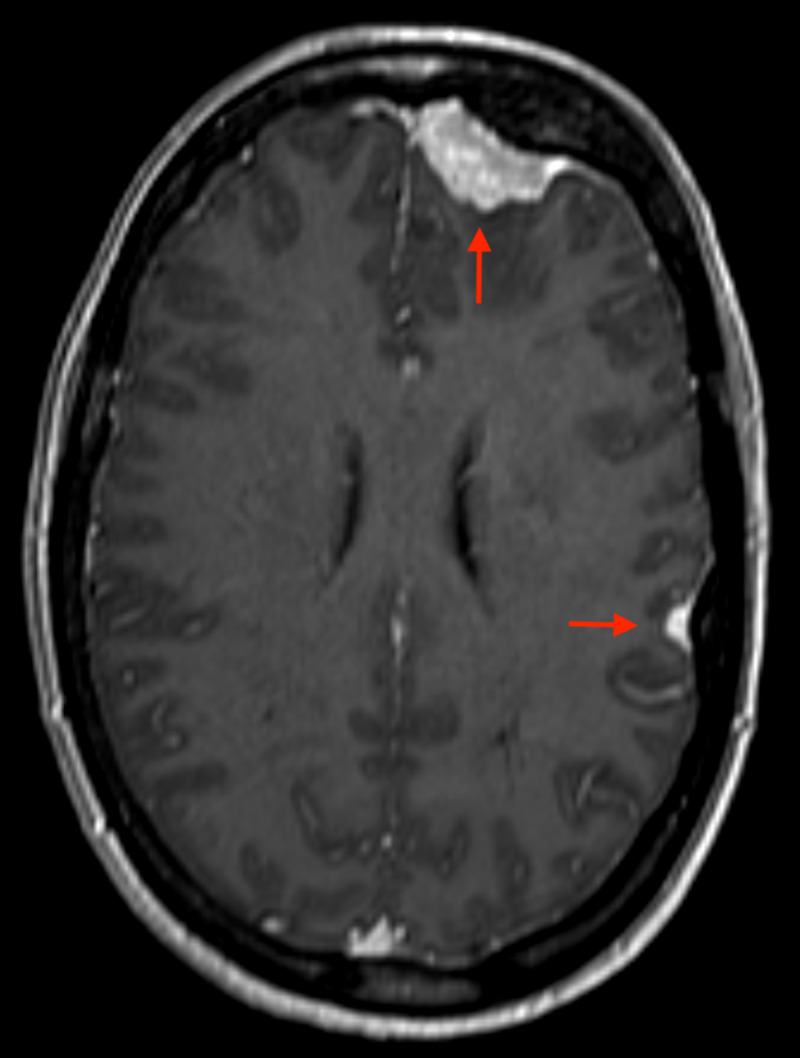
Axial T1-weighted MRI-contrast scan showing left frontal, and temporal meningiomata.
